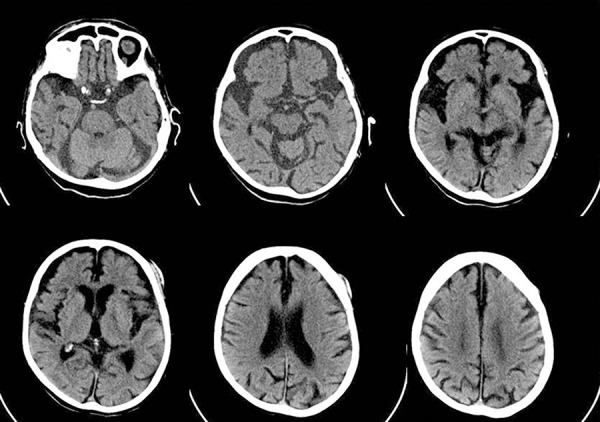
Brain computed tomography scan showing moderately severe atrophy in frontal
